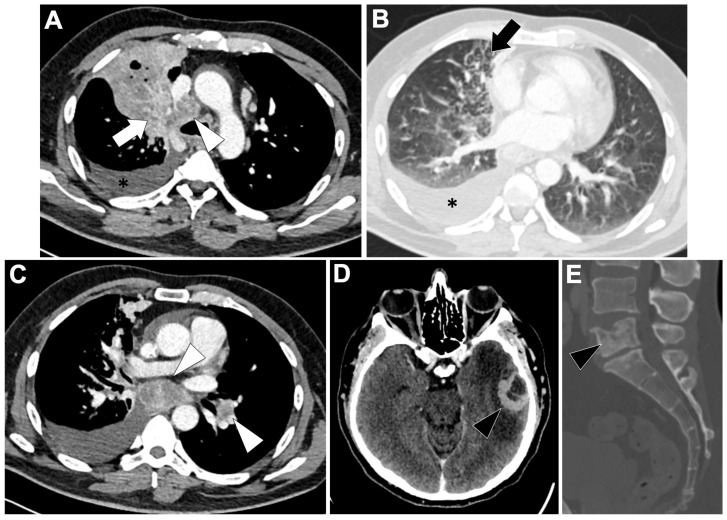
Representative baseline CT imaging findings in patients with ALK-altered lung adenocarcinoma (LUAD). (A) A 51-year-old never-smoking man presenting with a right middle lobe perihilar mass (white arrow) causing partial atelectasis, associated with right paratracheal lymphadenopathy (white arrowhead) and a pleural effusion (black asterisk). (B) Lung window images demonstrate peritumoral lymphangitic carcinomatosis (black arrow) in addition to the pleural effusion (black asterisk). (C) The CT scan also showed additional heterogeneous mediastinal lymphadenopathy, including subcarinal and contralateral nodes (white arrowheads). (D) Brain CT reveals a necrotic temporal brain metastasis (black arrowhead). (E) Bone window images show a mixed lytic–sclerotic metastasis involving the L5 vertebral body (black arrowhead).
